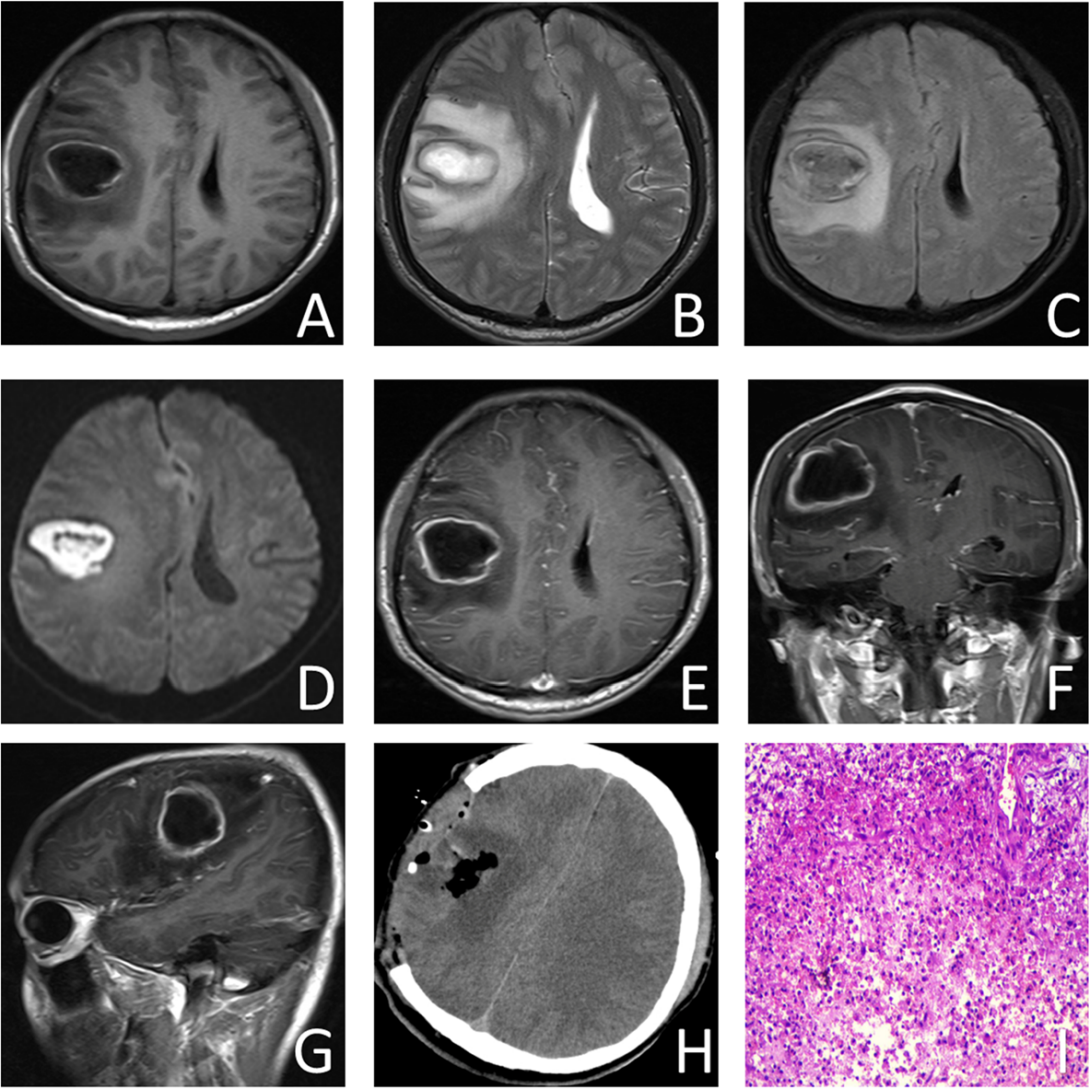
a–g Brain magnetic resonance imaging showed a 33 × 41 × 40 mm ring-enhanced capsule in the right frontal lobe with severe perilesional edema which leads to midline shifting to the left side, and the right ventricle was metamorphose. Diffusion weighted MR of the enhanced area revealed the lesion is an abscess. h Postoperative CT scan showed regular changes of post right temporoparietal craniotomy and deficiency of the right temporoparietal bone. i Pathological manifestation of the mass (HE× 100)
